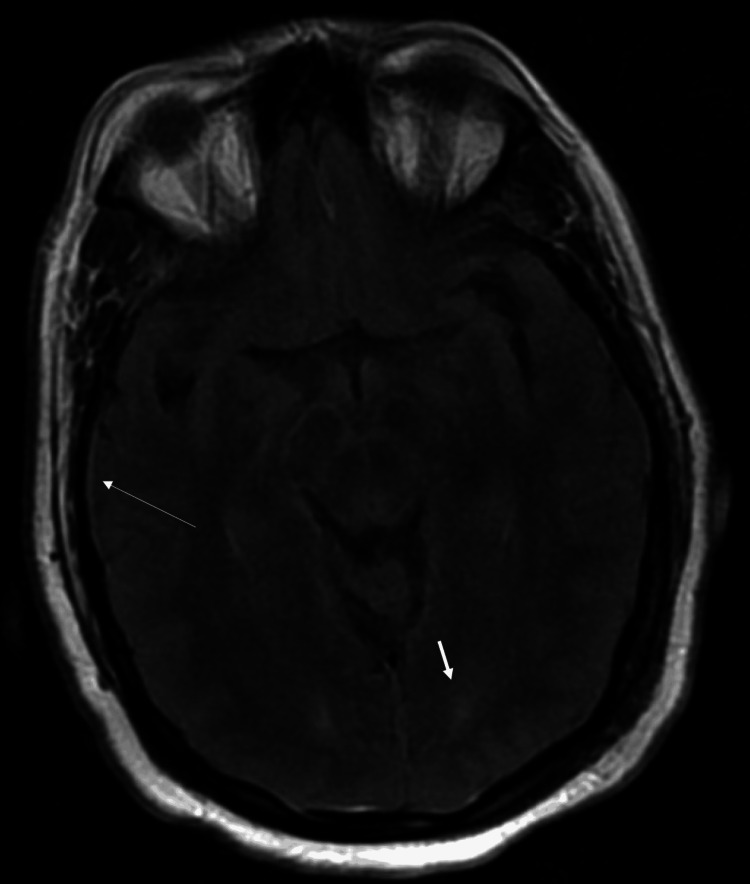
MRI of the brain w/ and w/o contrast T2-weighted MRI of the brain image with and without contrast reveals thickening of the dura mater (long arrowhead), suggesting inflammation. Additionally, nonspecific white matter hyperintensities are seen bilaterally in the parietal lobes (short arrowheads).
